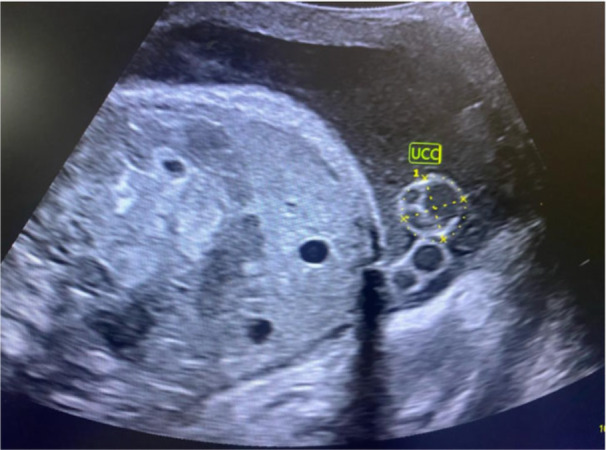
Sonographic measurement of umbilical cord circumference.
